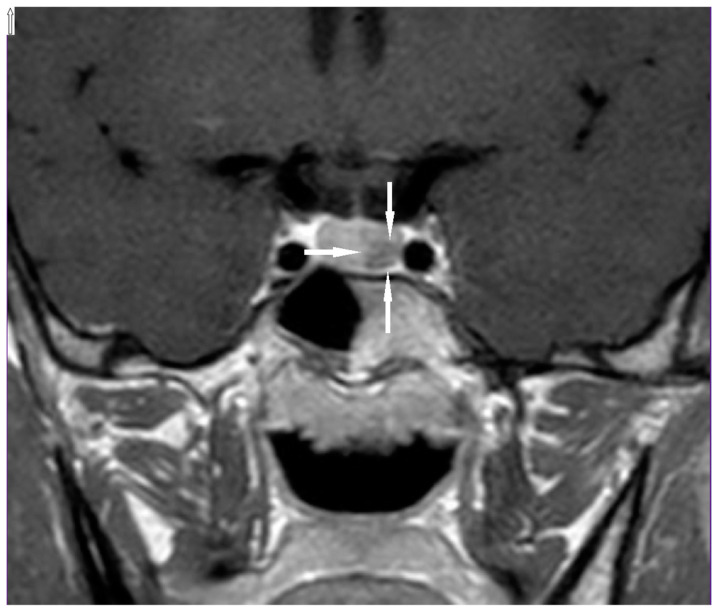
Pituitary microadenoma (arrows) visible in the MRI of the proband.
